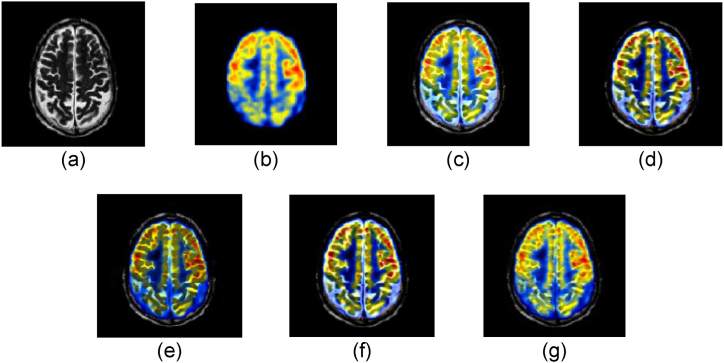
MRI and PET images fusion results of Alzheimer's disease, (a) MRI image, (b) PET image, (c) NSST-PAPCNN [24], (d) CNN [25], (e) CSMCA [26], (f) IFCNN [27], (g) Proposed.
